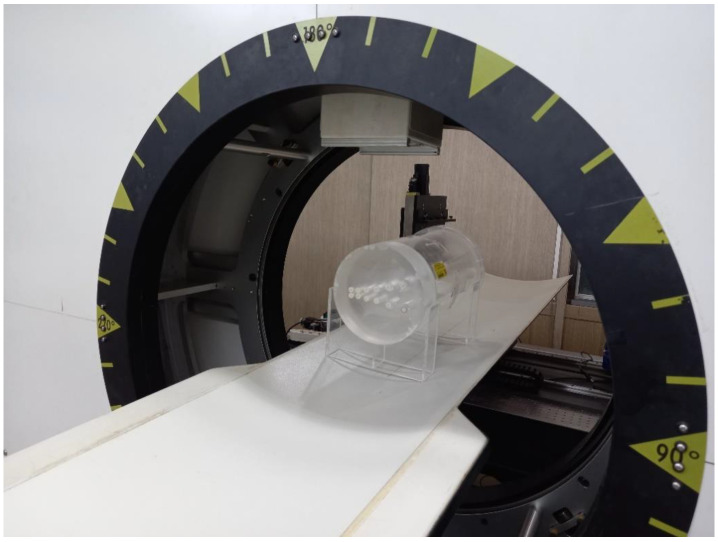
Photograph of the prototype cone-beam computed tomography (CBCT) system capable of both standard and ultra-high resolution (UHR) acquisition.
